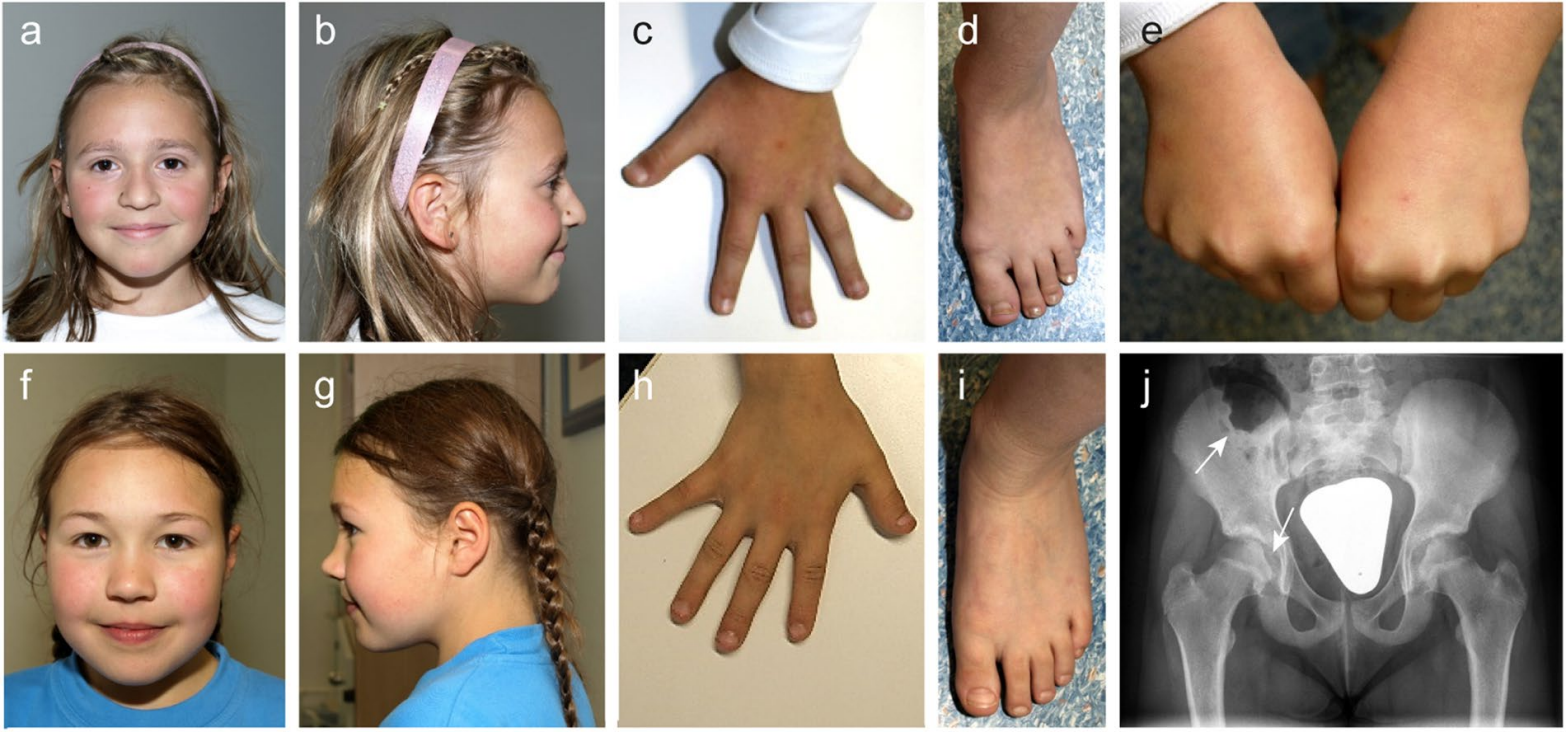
Clinical and radiographic characteristics of two patients with heterozygous ACAN mutations. (a–e) Patient P2 was born at term with a weight of 3270 g and a body length of 48 cm. At the age of 10 years she had a height of 119.6 cm (−3.6 SDS), weight of 25.2 kg (BMI 17) and head circumference of 50 cm (−1.6 SDS) (f–j) Patient P3 was born at term with a weight of 3550 g and a body length of 50 cm. At the age of 11 years she presented with a height of 121 cm (−3.9 SDS), weight of 28.4 kg (BMI 19) and head circumference of 50 cm (−1.7 SDS). Psychomotor development was normal in both patients. Both presented with proportionate short stature, broad chest, short neck, brachydactyly with pronounced brachymetacarpaly V, broad thumb and great toe and an inhibition of extension of the elbow. (a,b,f,g) Facial anomalies include a broad nasal tip and a high forehead. (j) Radiographic evaluation revealed signs of Osteochondritis dissecans (arrows) in patient P3, her mother and maternal grandmother.
